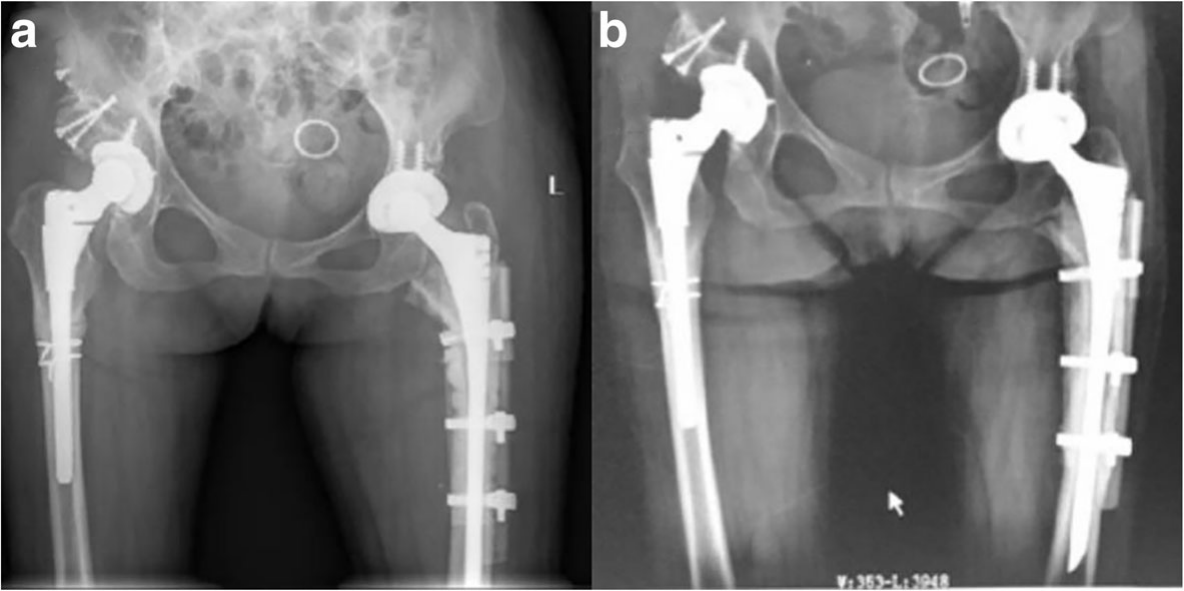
Radiographic images after revision surgery (a-b). a Radiograph of the patient’s left revision THA 1 day postoperatively showed that the prosthesis was fixed. b Radiographic image showed that the subtrochanteric osteotomy of the left femur is healed at a 12-month follow-up after the patient’s revision surgery
